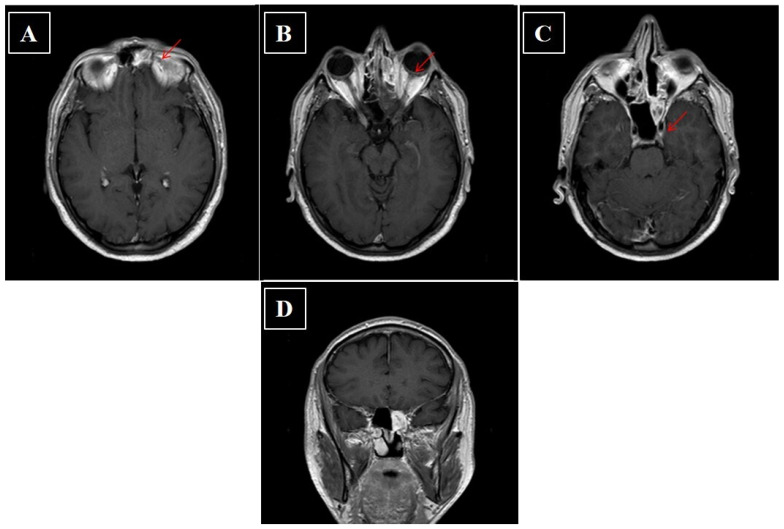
(A–D) Axial and coronal contrast enhanced fat-suppressed T1-weighted image CT scan of head performed after surgery. The arrow indicates persistence of inflammatory tissue.
